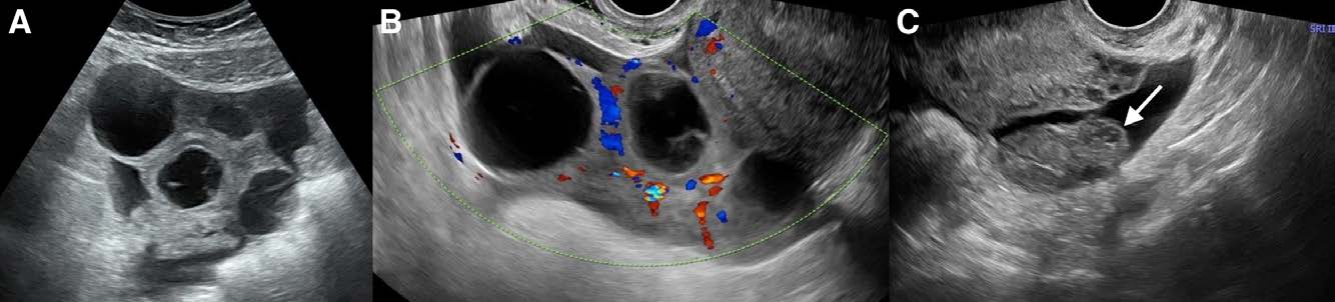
(A) Two-dimensional transabdominal ultrasound image showing enlarged right ovary with multiple thin-walled cysts. (B)Transvaginal color Doppler ultrasonography showing prominent peripheral blood flow surrounding the cyst, displaying a characteristic “spoke wheel” sign. (C) Normal-size left ovary (indicated by the arrow).
